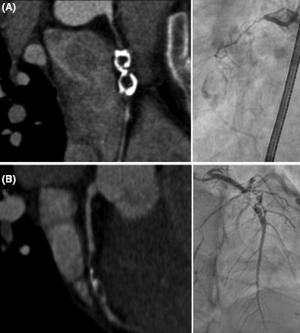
Coronary computed tomography angiography and coronary angiography showing a giant calcified aneurysm of the right coronary artery (RCA) (A) and a severe stenosis in the mid segment of left anterior descending artery (LAD) (B)
